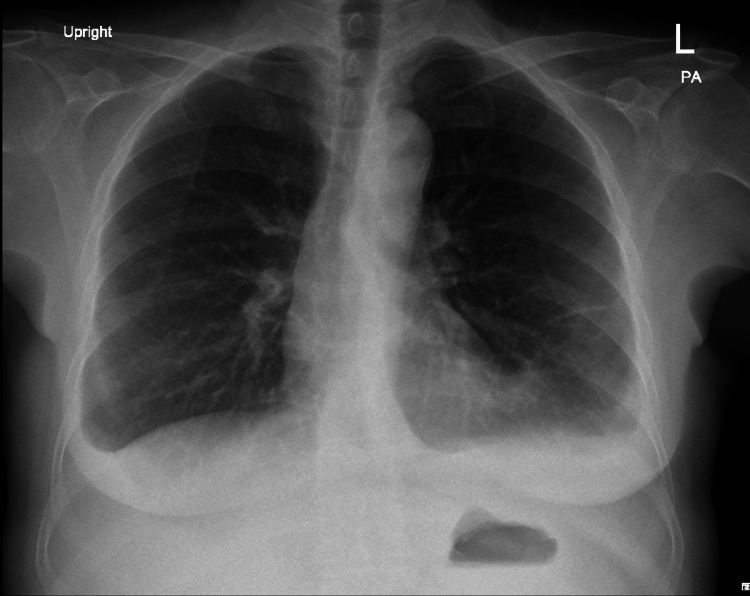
Chest X-ray showing blunting of bilateral costophrenic angles-mild pleural effusion.
